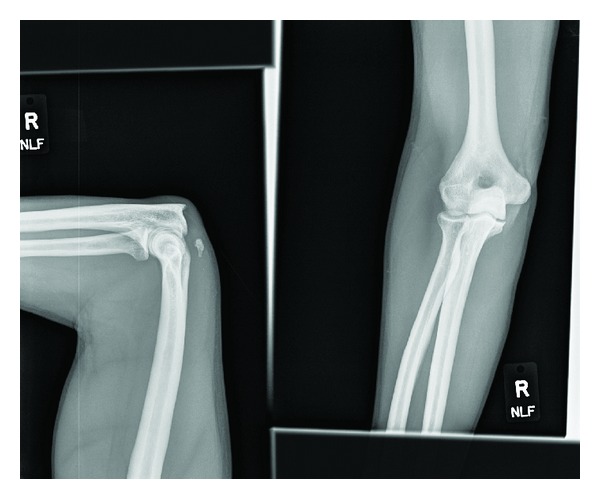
Plain radiograph, right elbow with avulsion fracture.
